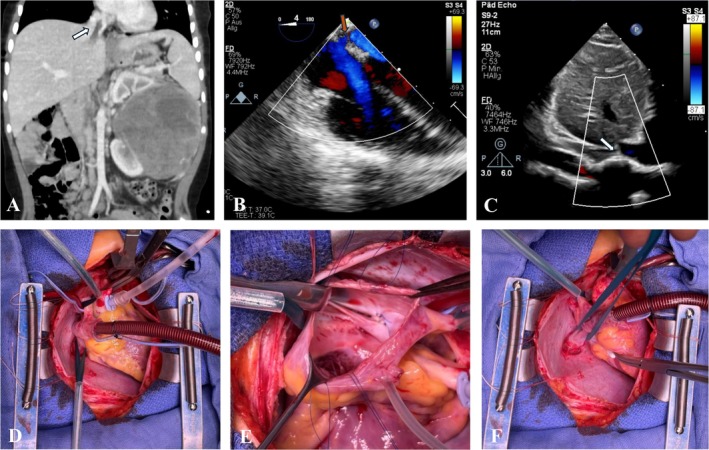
(A) CT‐scan showing the left‐sided nephroblastoma and the inferior vena cava thrombus (white arrow). (B) Transesophageal echocardiography showing the PFO (red arrow). (C) Transesophageal echocardiography showing the inferior vena cava thrombus (white arrow). (D, E, F) Intraoperative views of the PFO closure under cardiopulmonary bypass and circulatory arrest.
